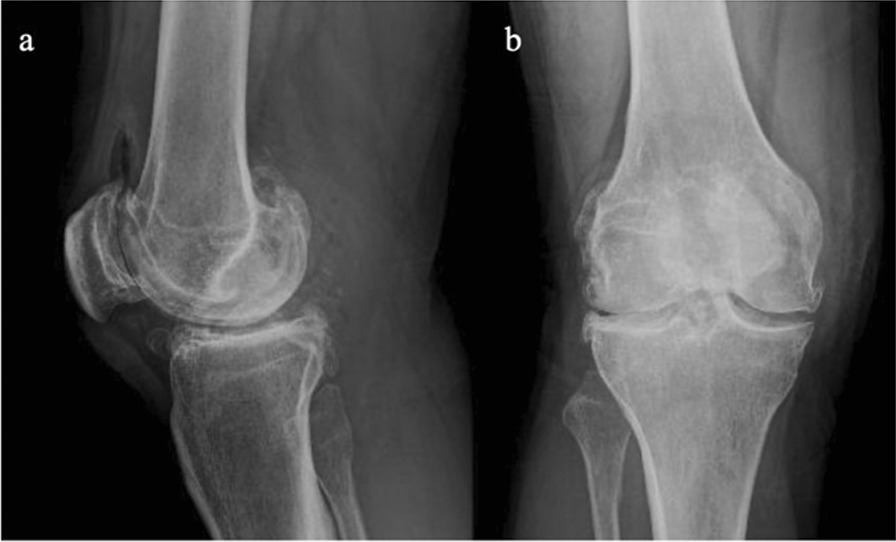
a Anterior–posterior and b lateral postoperative radiograph
